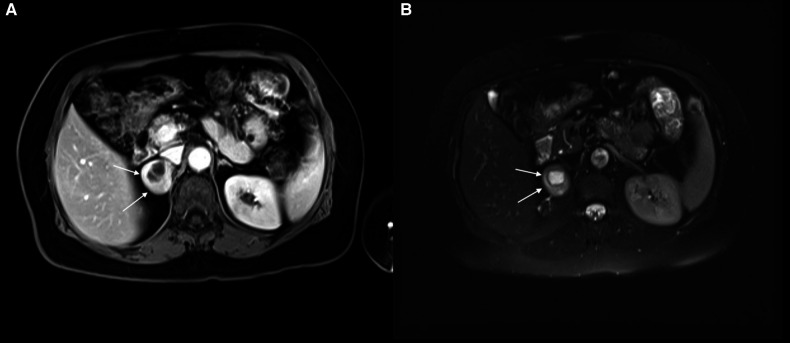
(A) right adrenal schwannoma in the T1 sequence of MRI. The cystic necrotic component can be seen in this imaging study. The schwannoma was shown by a white arrow. (B) Right adrenal schwannoma in the T2 sequence of MRI. The schwannoma was shown by a white arrow.
